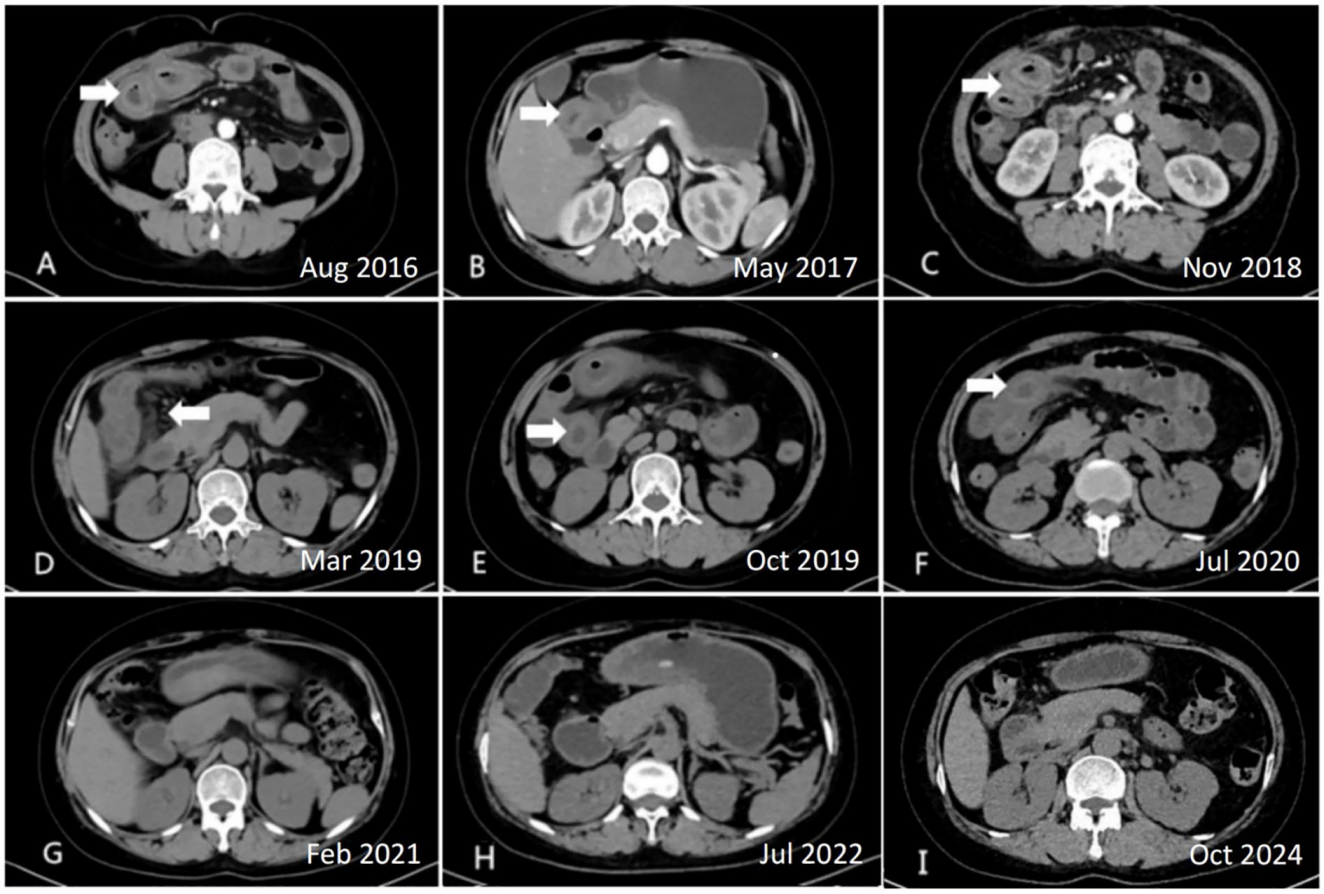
Serial abdominal computed tomography findings in lupus mesenteric vasculitis (LMV) before and after belimumab treatment. (A-F) Active LMV flares demonstrating bowel wall thickening,”target sign,” and “comb sign” (2016–2020); (G-I) Normalized bowel wall post-belimumab (2021–2024).
